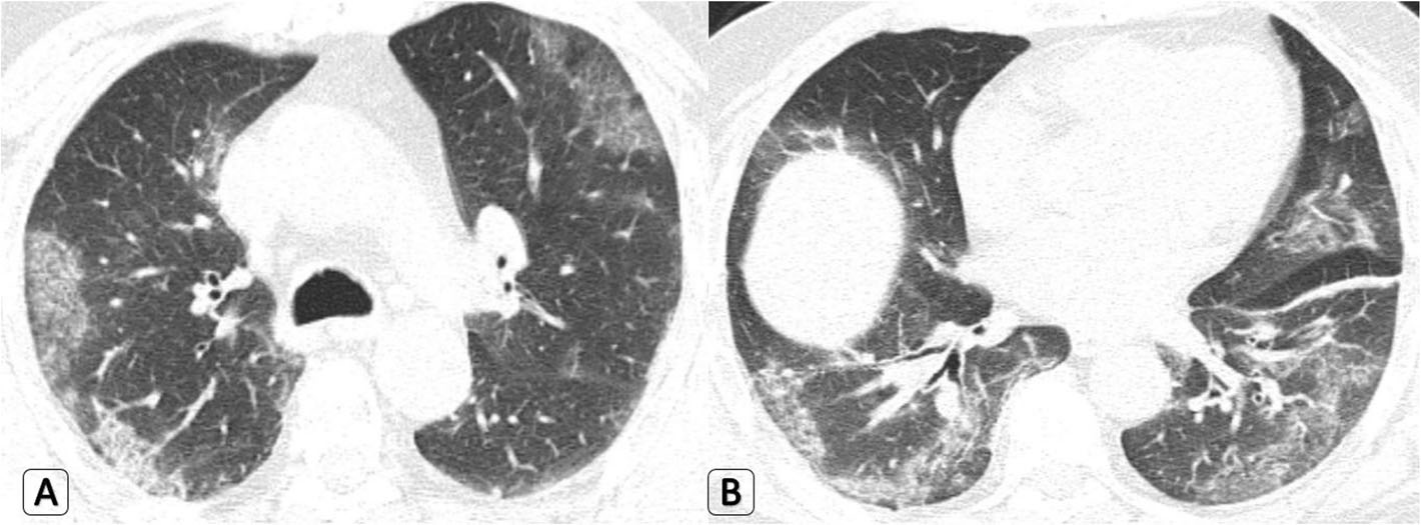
A 45-year-old male patient who had positive contact history with recently proved COVID-19 patient and was completely asymptomatic. a-b Axial HRCT chest lung window showed bilateral large peripheral located ground-glass patches with mild septal thickening (evolving crazy paving pattern) and left basal fine atelectatic band. Clinical decision was home isolation, medical treatment, and PCR testing. Positive PCR result for COVID-19 was proved later
